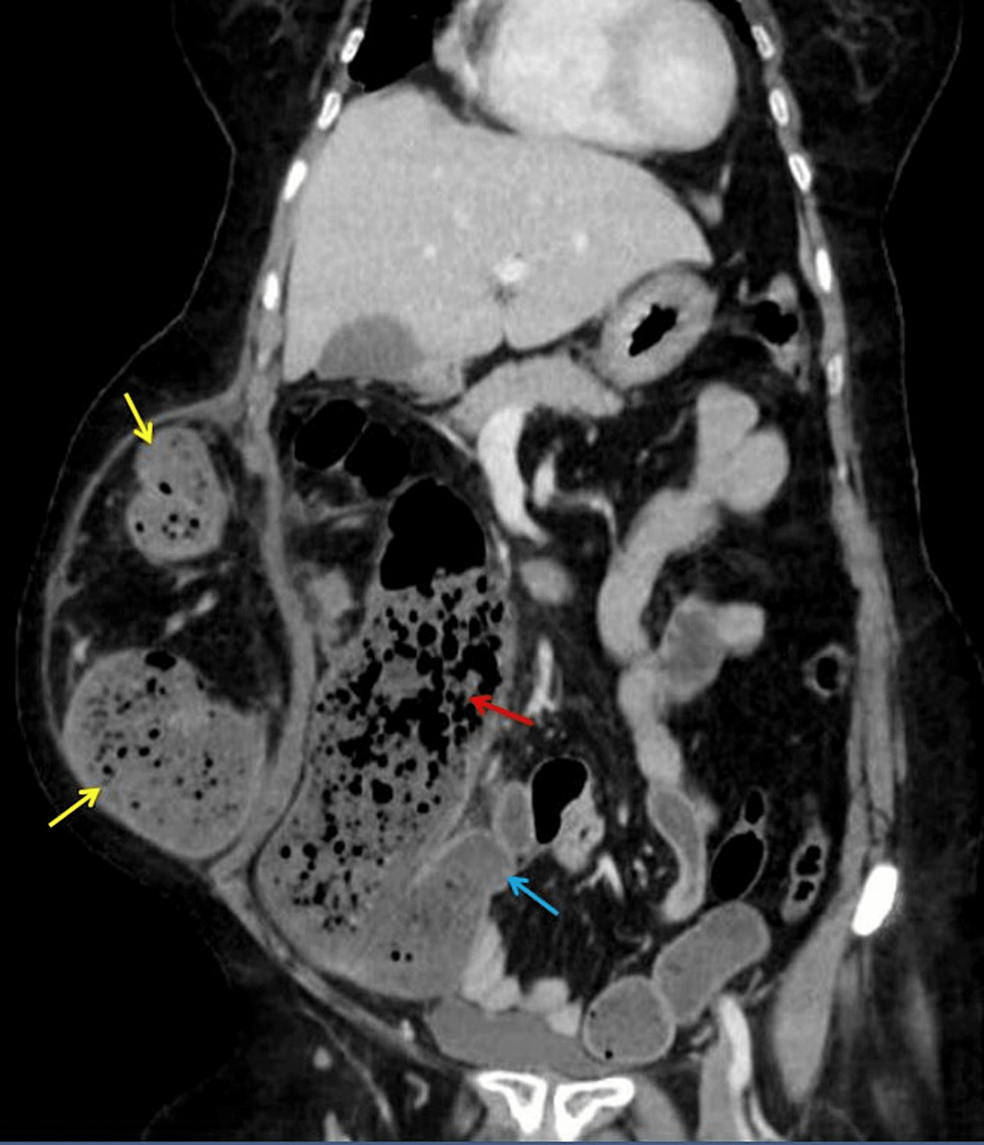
Computed tomography (coronal view) showing grossly dilated right colon (red arrow), the ileocecal junction (blue arrow), and herniated transverse colon volvulus at the previous drain site (yellow arrows).
